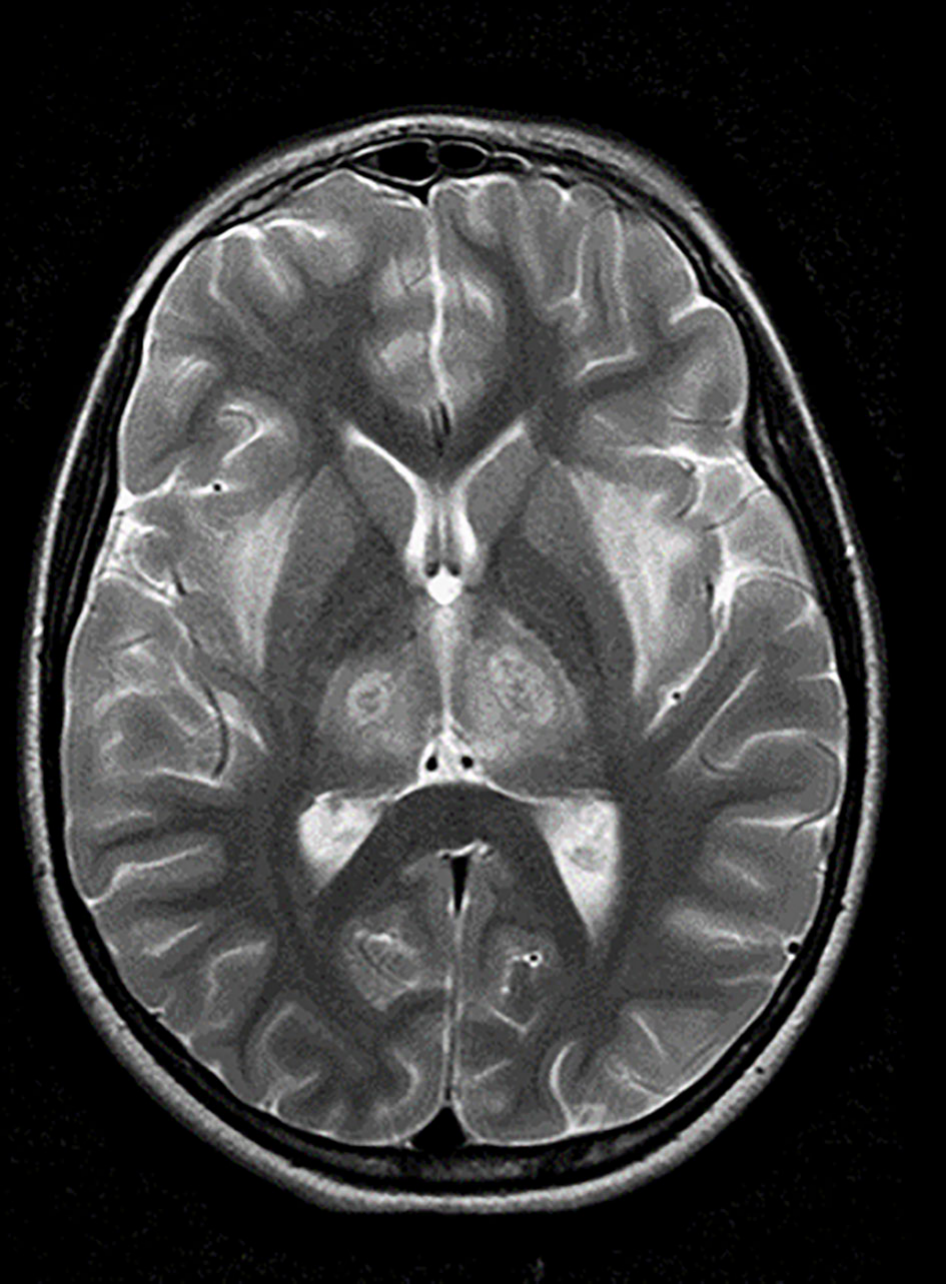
Acute Necrotizing Encephalopathy (Local case). (Personal case of APN and JMW): Axial T-2 weighted MRI of a 9-year-old girl with who presented with classical clinical features of ANE and was admitted to the local paediatric intensive care unit. The MRI shows the classical symmetrical involvement of both thalami (with a target appearance) and symmetrical external capsular white matter affected. She had brainstem involvement (not shown) and was treated with intravenous methylprednisolone early. She survived with mild to moderate neurological sequelae. She was the first in her family to be genetically confirmed as positive for a RANBP2 mutation, with two of her cousins having been previously affected. The genetic result assisted with identification of at-risk family members, counseling and subsequent preventative measures including vaccination and early ANE ‘crisis’ management.
